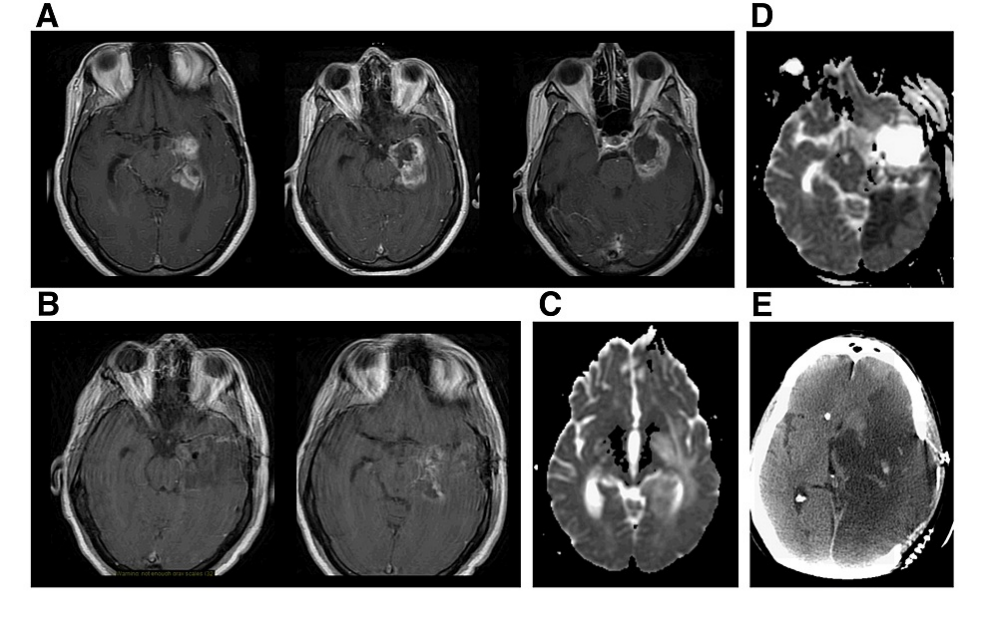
Neuroimaging of delayed ischemia Preoperative (A) and postoperative (B) axial gadolinium-enhanced T1-weighted MR images showing a recurrence of the temporal glioma around the edges of the previous resection cavity and partial resection with a thin layer of tumor remaining in the medial temporal lobe. Postoperative DWI (C) shows no evidence of ischemia. Eight days following surgery, DWI shows acute blood in the resection cavity while CT imaging shows a dense PCA (D). Two days following this scan, imaging revealed a large MCA infarction (E). DWI: diffusion-weighted imaging; PCA: posterior cerebral artery; MCA: middle cerebral artery
